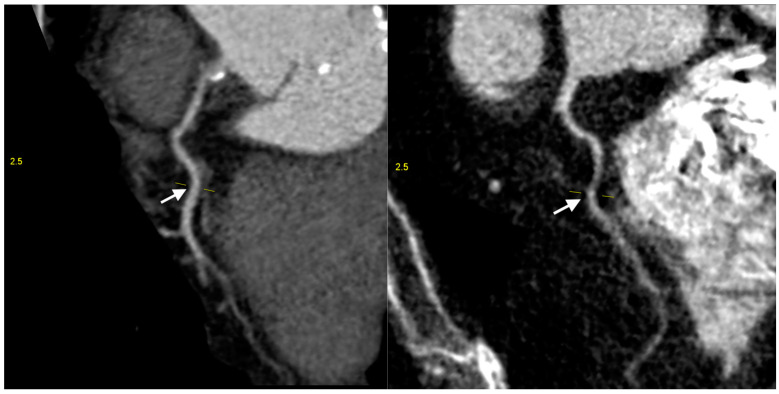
Coronary computed tomography angiography of hypoplastic right coronary arteries (white arrow) (images are upscaled to a 2.5 factor).
